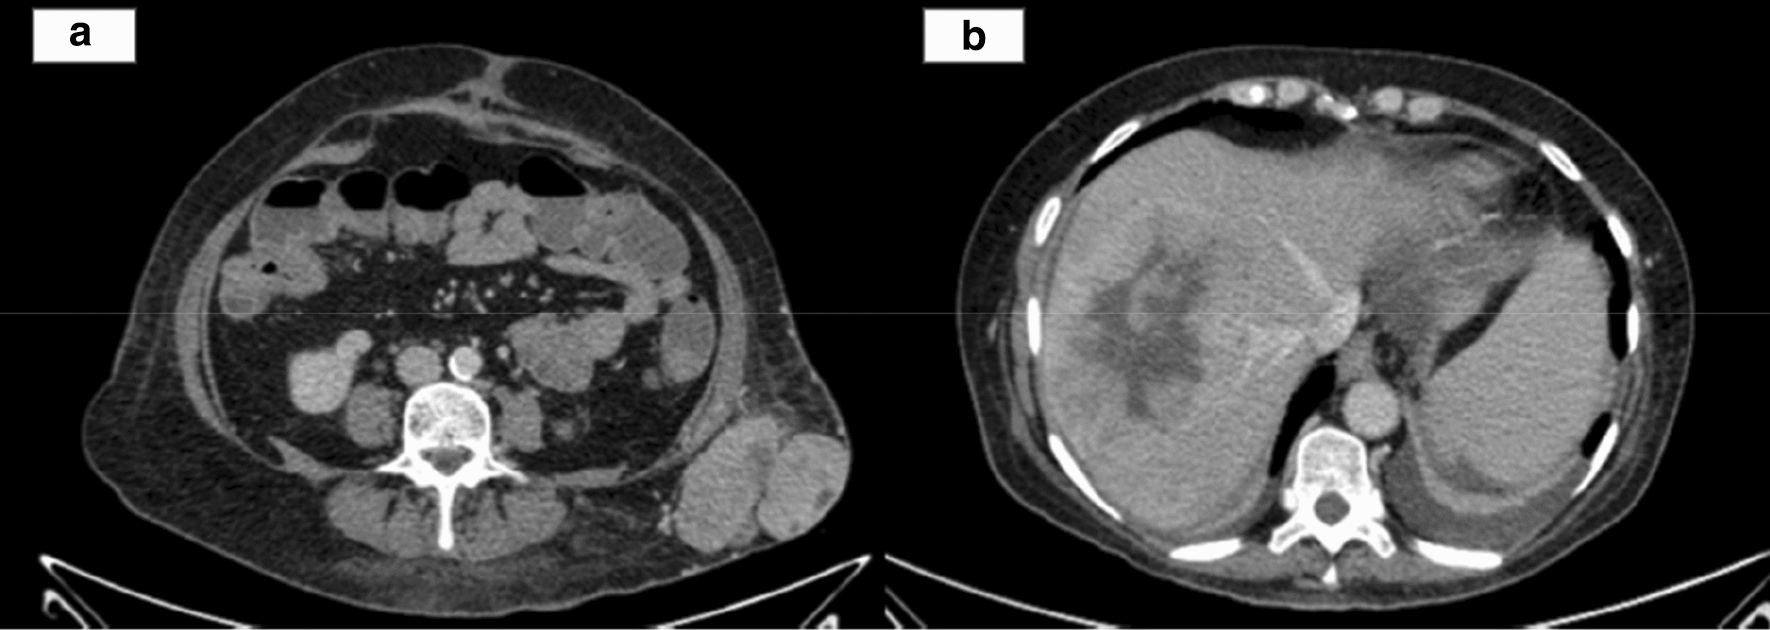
CT scan (a) primary tumour in left flank. b Giant liver metastases
